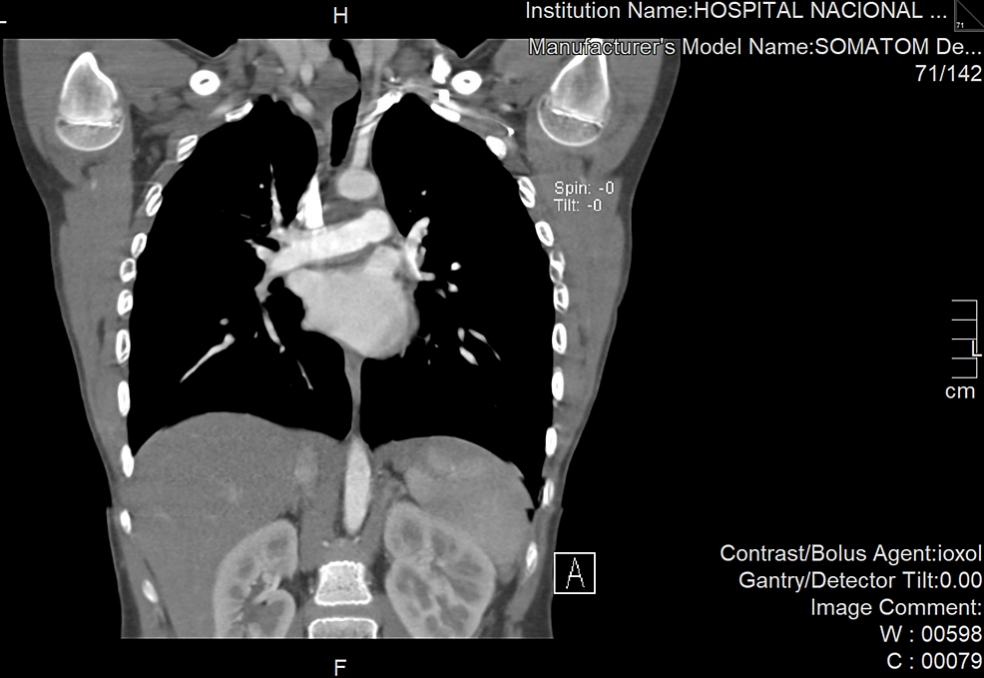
Chest CT A 16x22x30 mm heterogenous mediastinal mass at the right posterolateral level of the trachea
